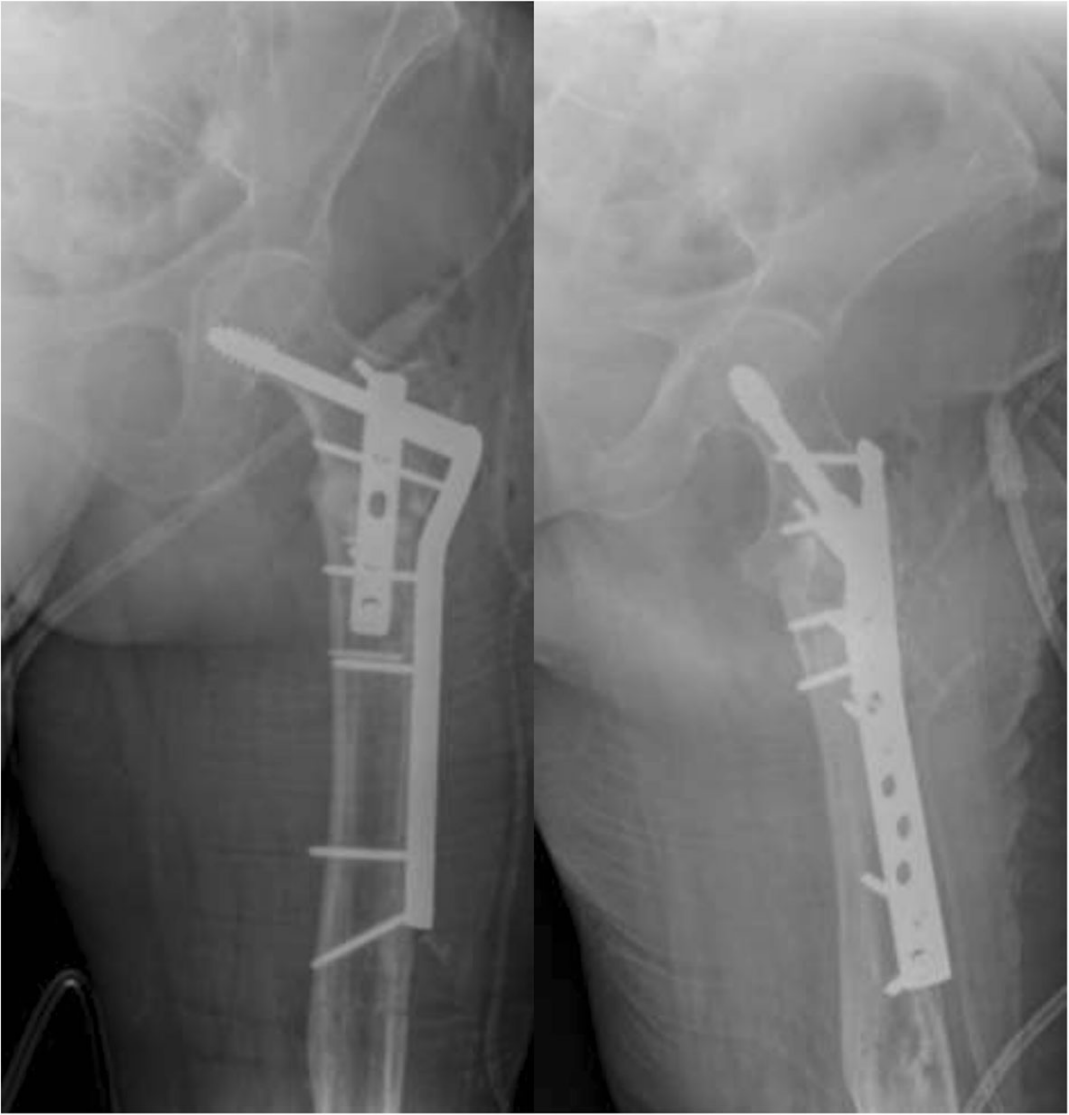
Postoperative X-rays after nail removal, restoration of the CCD (126°) and DCS treatment with a precontoured plate by leaving the augmentation plate in situ
